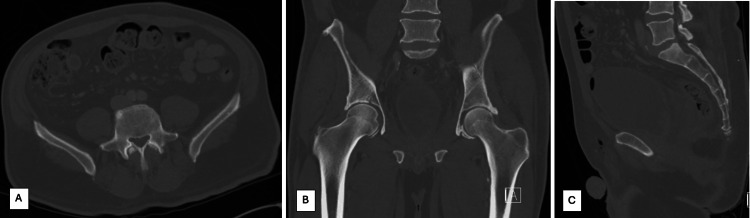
Non-contrast computed tomography of the pelvis performed around the time of the patient's prostatectomy, which did not demonstrate any abnormal osseous lesions. Limited visualization of the L4 vertebral body given the scan had been ordered as a "CT pelvis." Figure 1A demonstrates an axial cut through the superior aspect of the L5 vertebral body, with no lesion evident. Figure 1B demonstrates a coronal cut, allowing for the visualization of the mid-portion of the L5 vertebral body without any osseous lesion identified. Figure 1C demonstrates a sagittal cut, allowing for the visualization of the mid-portion of the L5 vertebral body, also with no lesion noted.
